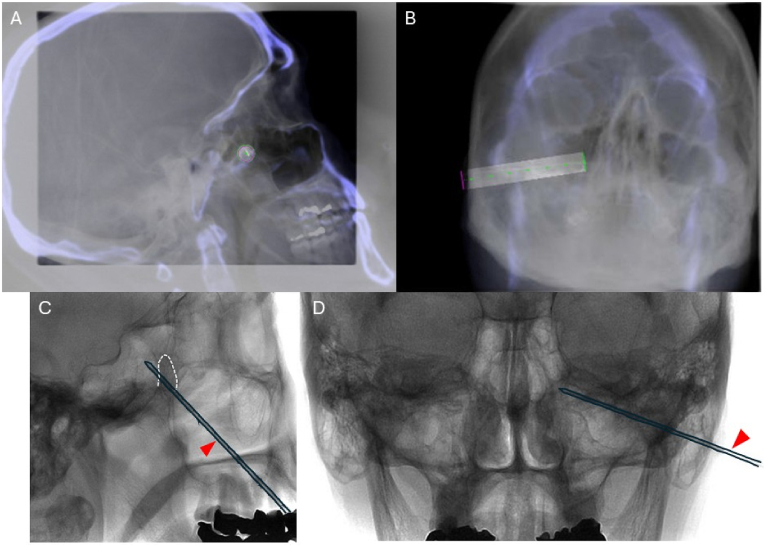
A) In the entry view of the angiocath, a 2D fluoroscopy image is superimposed on the reconstructed cone beam–based CT (CBCT) imaging, depicting the angiocath being advanced coaxially along the planned trajectory toward the pterygopalatine fossa. The tail of the angiocath is in overlap with the tip of the needle, so the angiocath axis appears as a single overlapping green/pink circle on the background 3-D CBCT imaging. B) In the progress view, the shaft of angiocath is in overlap with the planned trajectory in green line and the tip of angiocath stopped at the target pterygopalatine fossa. C) In lateral view, the final position of angiocath was confirmed with tip within pterygopalatine fossa. White dashed outline: pterygopalatine fossa, red triangle: 14g 3 1/2″ blunt angiocath. D) In posterior-anterior view, the final position of angiocath was confirmed with tip at the level of middle turbinate of lateral wall of nasal cavity. (For interpretation of the references to colour in this figure legend, the reader is referred to the Web version of this article.)
